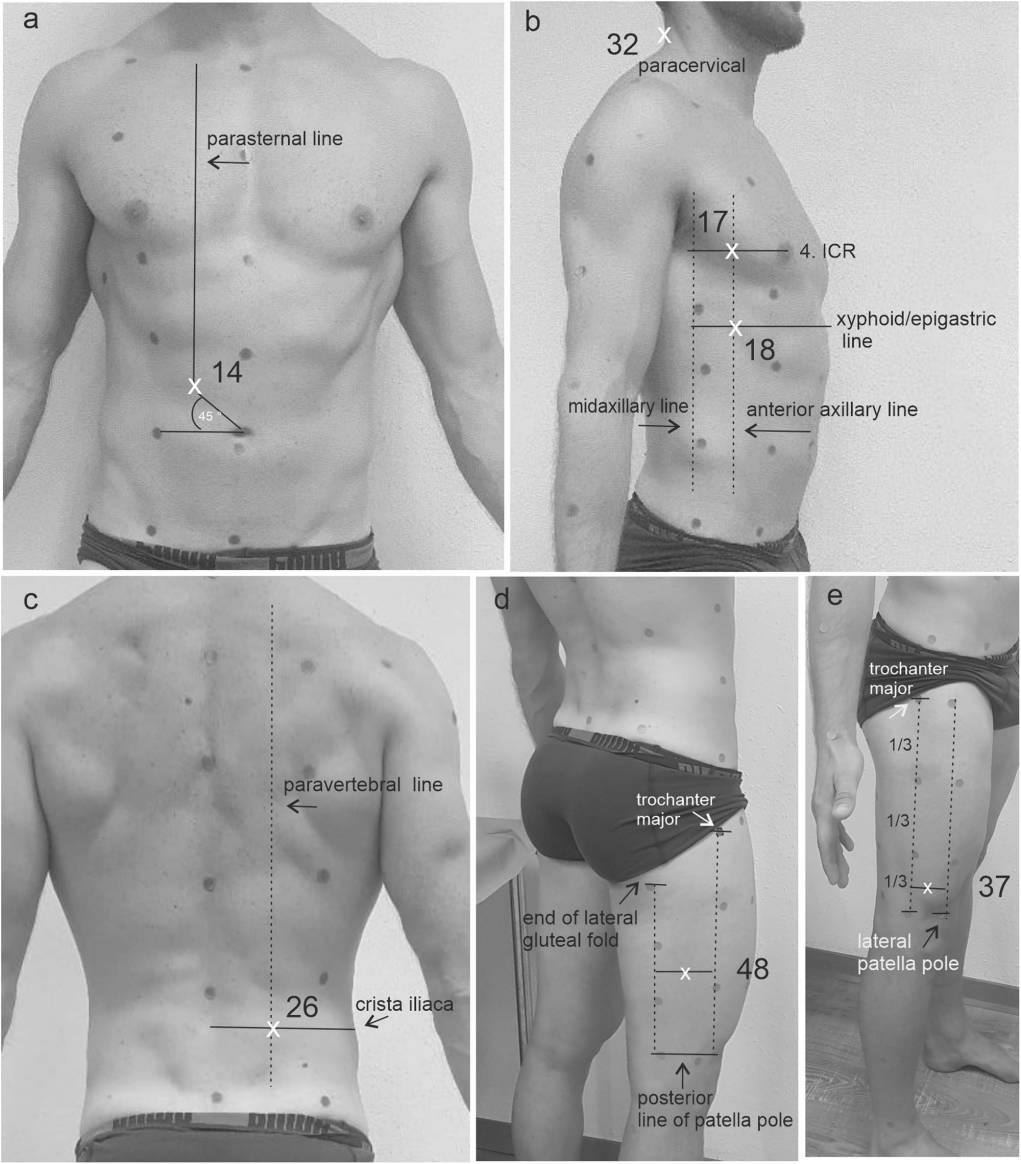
VAT_Eq_ landmarks. a Intersection of parasternal line and a 45°line at umbilicus creates F14. b Paracervical landmark at midcervical spinal (F32); Intersection of anterior axillar line with horizontal 4. ICR line (F17) and xyphoid/epigastric line (F18). c Intersection between paravertebral line and posterior, horizontal line of cristia iliaca. d One orientation point is identified at half distance of the vertical line from end of lateral gluteal fold to the horizontal posterior upper patella pole line. This orientation point creates a horizontal line ending at the vertical trochanter major line, where F48 is identified at the center. e The distance of vertical trochanter major line ending at the posterior patella pole line forms three equal sizes or lengths. The last third constitutes a point in its center, which is horizontally extended to the vertical patella pole line. The center of that line forms the measurement point (F37). All landmarks are designed for the body’s right side.
